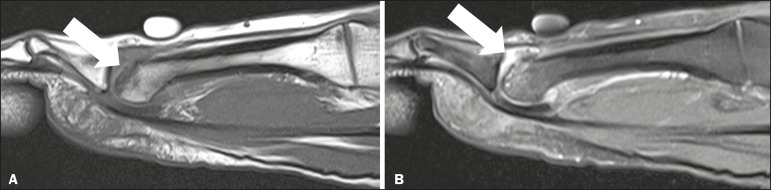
T1-weighted and fat-saturated T2-weighted MRI sequences showing
